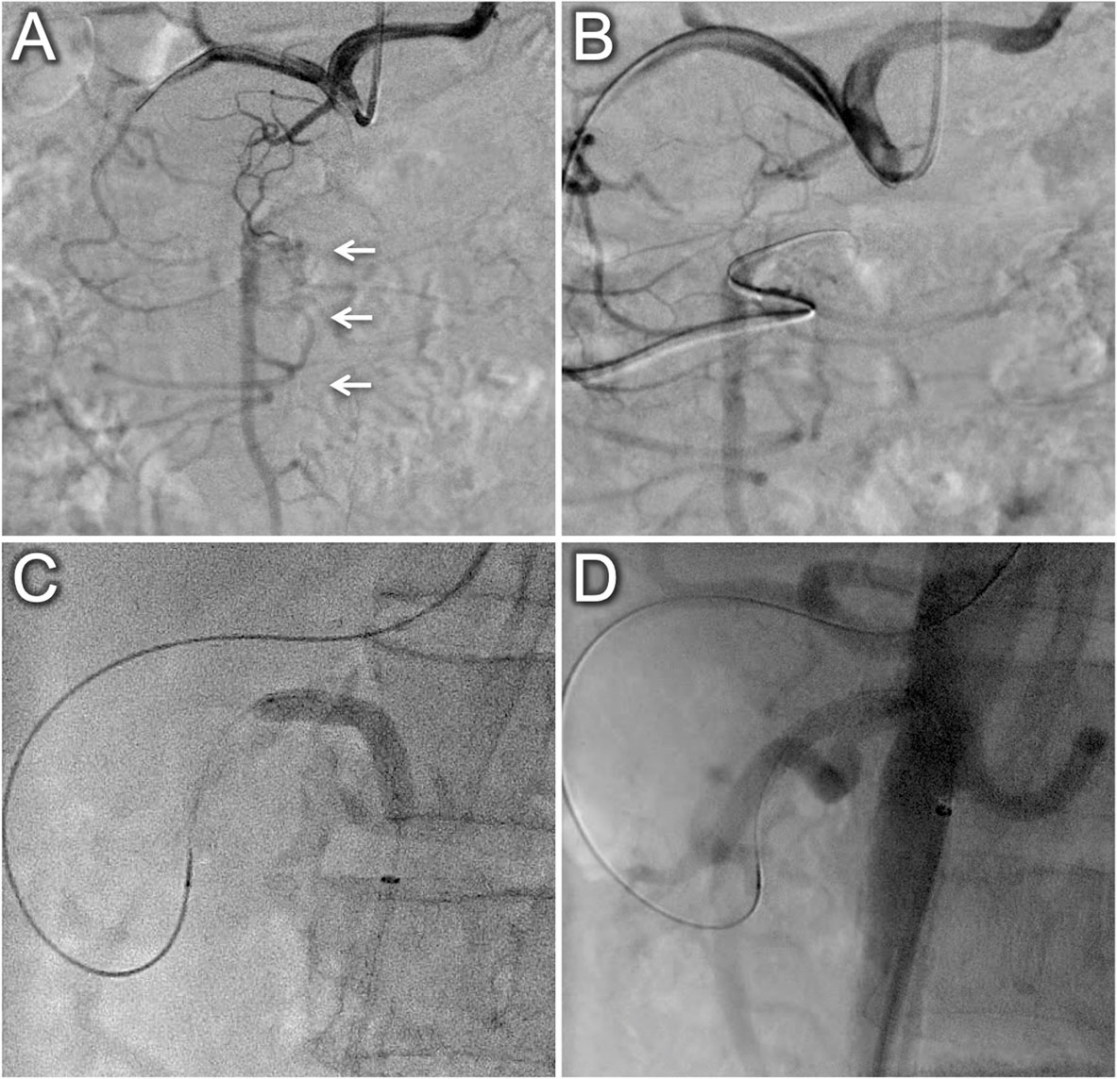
Angiography and endovascular therapy. A, Digital subtraction angiography from the celiac artery showed collateral blood flow supplying to the superior mesenteric artery (SMA) (white arrows); however, did not show the pancreaticoduodenal arcade clearly. B, The Jupiter SFC guidewire with Corsair microcatheter reached the distal portion of the chronic total occlusion (CTO) in the SMA through the pancreaticoduodenal arcade as shown by computed tomography imaging. C, A 6.0 mm balloon was dilatated in the CTO. D, Angiography showed antegrade blood flow in the SMA after two balloon expandable stents
